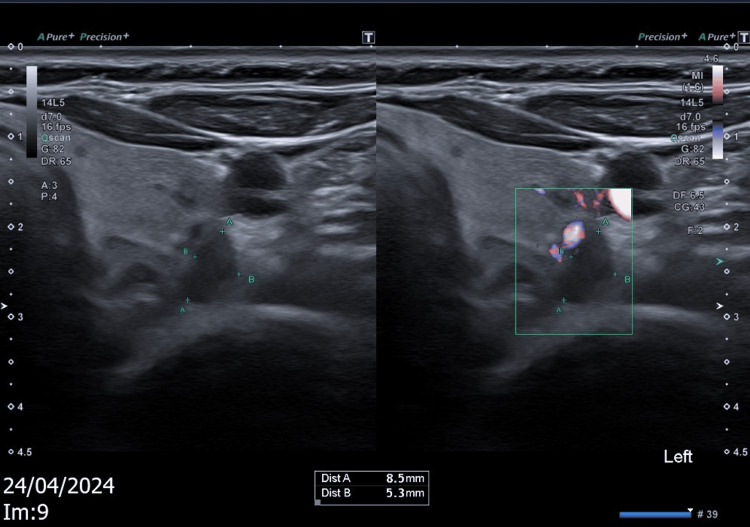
Neck ultrasound showing 9 mm hypoechoic lesion posterior to the lower pole of the left thyroid.
